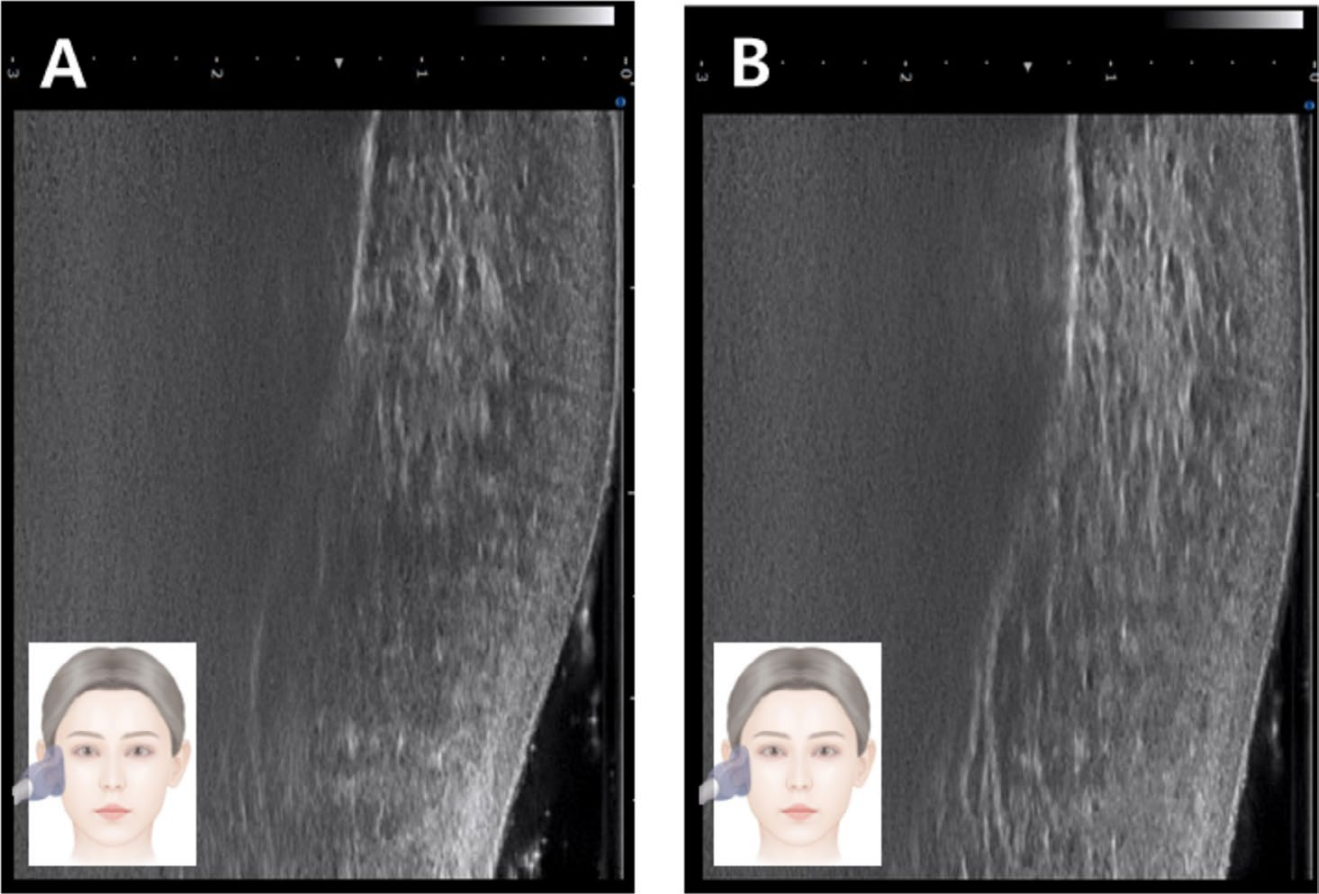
Vertical ultrasonographic image of the zygomaticus ligament proper before (A) and after (B) treatment.
